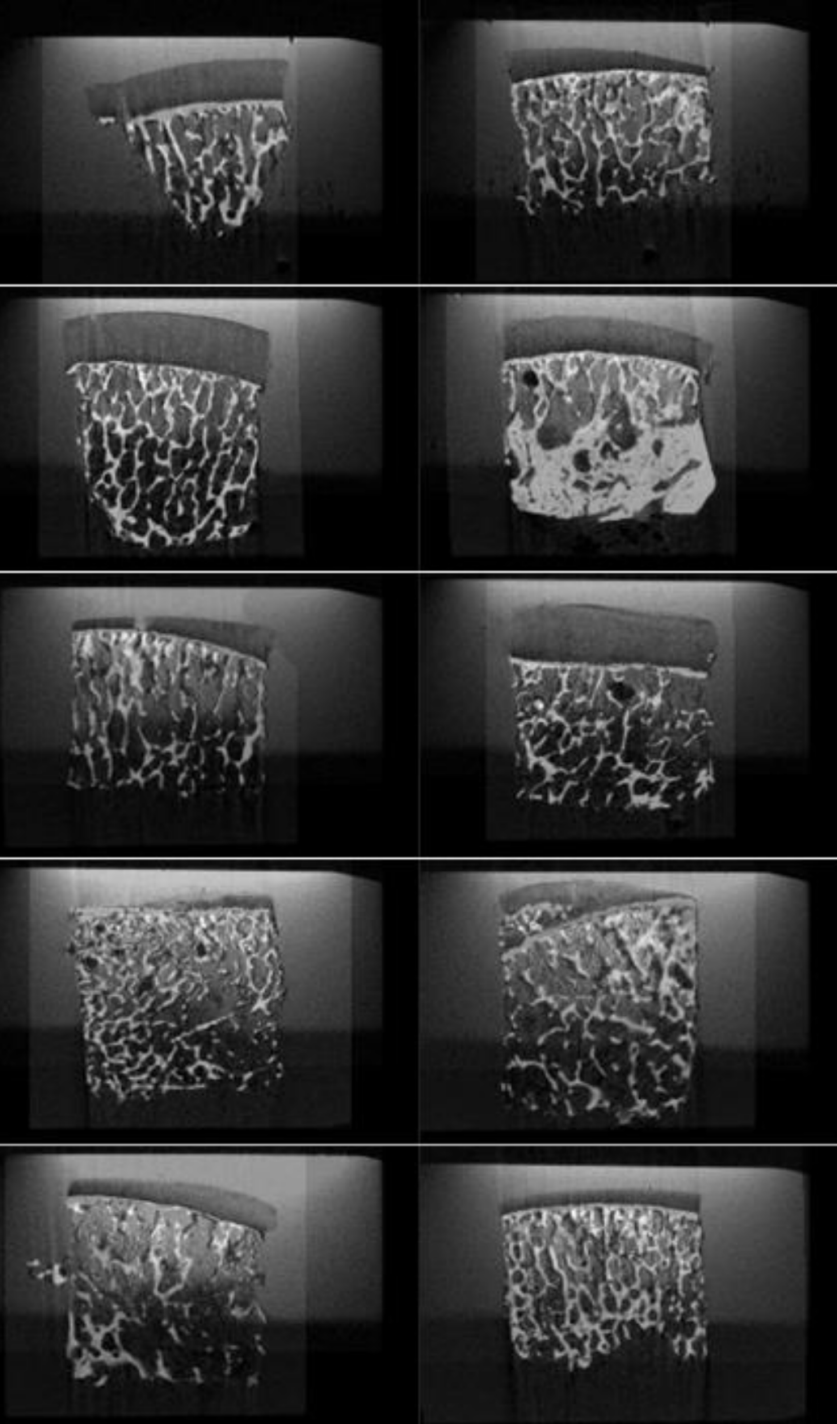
The final alignment of micro-computed tomography and micro-magnetic resonance imaging scans of all 10 samples.
